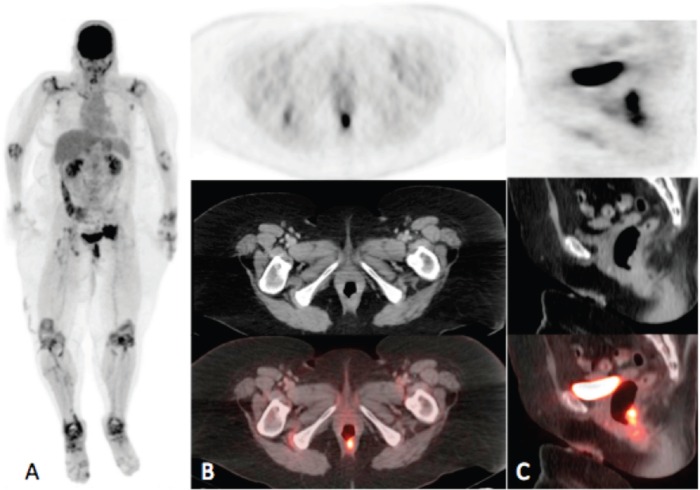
Maximum intensity projection (MIP) (A) and PET, CT and fusion images in the axial (B) and sagittal (C) planes. MIP image demonstrates intense uptake in the rectum and in the pelvis to the left of the urinary bladder. Axial and sagittal images demonstrate intense uptake (SUVmax = 17.4) localising to a rectal soft tissue mass. Note on the MIP image symmetric bilateral circumferential hyper-metabolism surrounding the joints in the upper and lower extremities consistent with the known history of rheumatoid arthritis.
